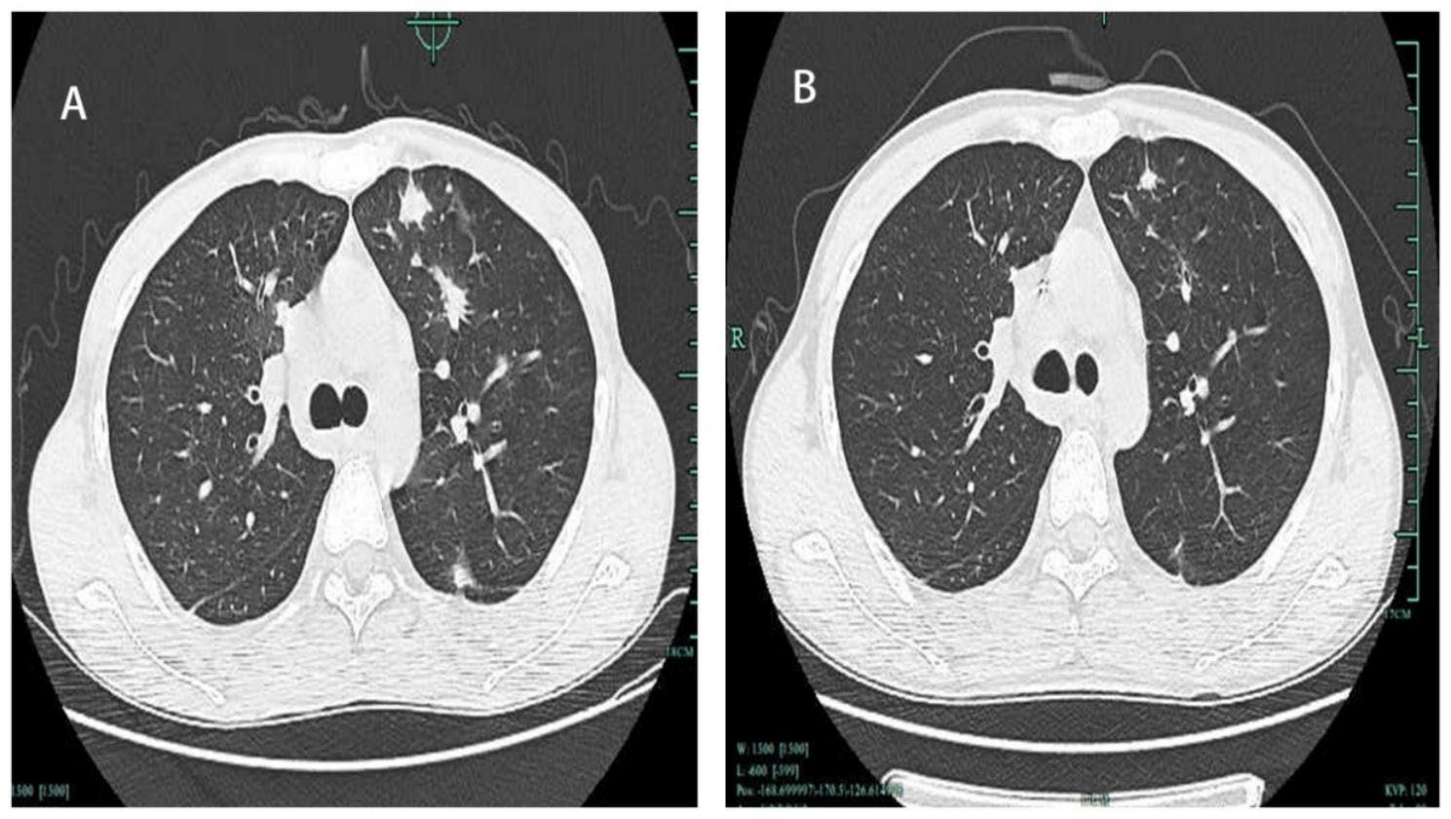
Computed Tomography (CT) of the lungs showed many small patches, nodules, and patchy high-density shadows, with blurred boundaries and uneven density (A). After six months of treatment, CT of lung condition improved (B)
